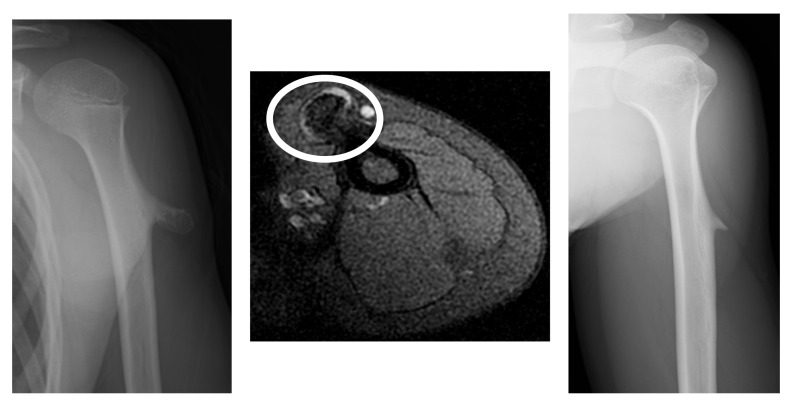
Radiographs revealing a pedunculated-type osteochondroma of the proximal humerus (left). At initial diagnosis, the thickness of the cartilage cap is 2.25 mm (circle) on MRI (middle). Two years later, regression of osteochondroma was observed (right).
